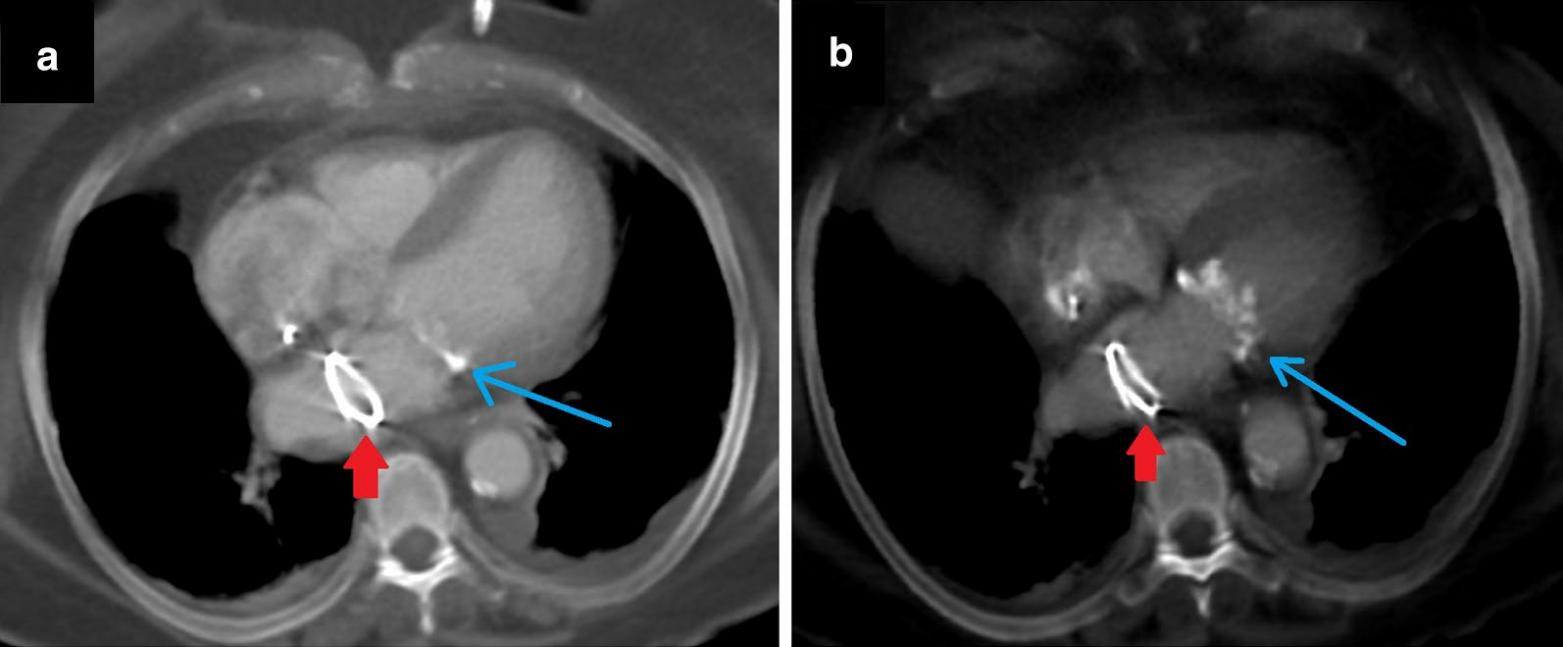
Importance of effective communication. A 65-year-old female patient suffered from the new onset of dyspnea three days after mitral valve replacement. Thorax CT showed the intra-atrial location of the replaced mitral valve (red arrows, a, b). When the abnormal valve location and new onset of dyspnea were considered, displacement of the replaced mitral valve was suspected. The cardiovascular surgery department was immediately called to report this situation. After a brief opinion exchange with the referrer, it was learned that this valve location is the expected location in patients who have undergone mitral valve replacement with the Chimney technique, which is preferred for patients with extensive mitral annular calcifications (blue arrows, a, b) to prevent calcified embolism during the procedure. As a result, effective communication between the radiologist and the clinician overcame the possibility of “lack of knowledge and overreading errors”
